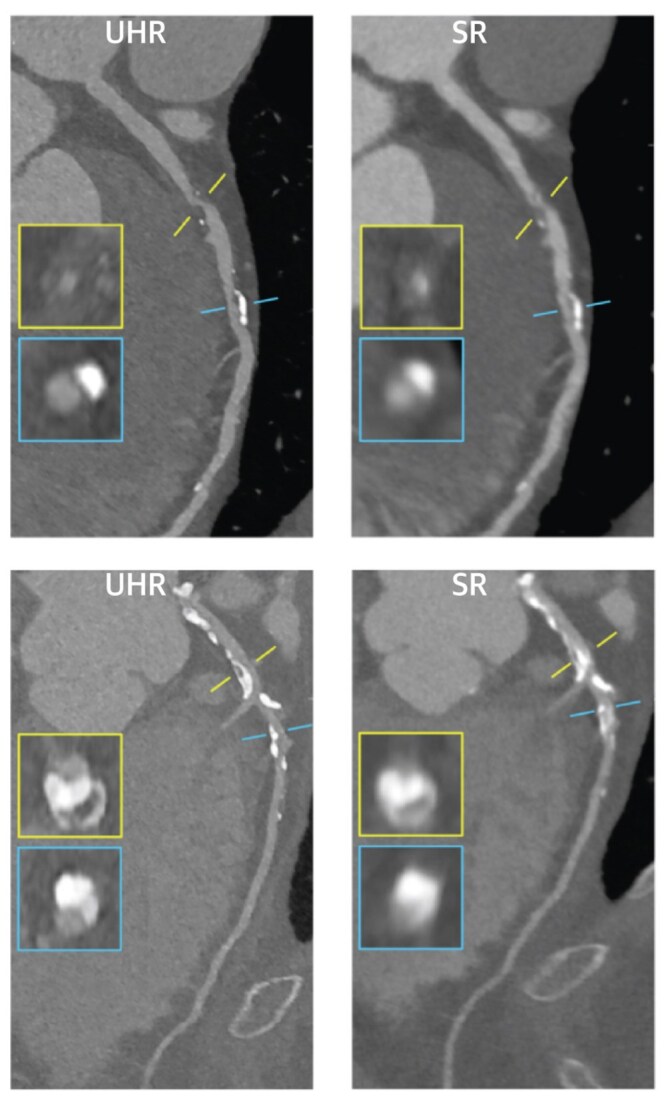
The left anterior descending artery in two patients (upper and lower) demonstrating differences in image readability between ultra-high resolution vs standard resolution photon counting detector CT scans. Ultra-high resolution (UHR); standard resolution (SR). Adapted and reproduced from Kotronias et al.^13^ under a creative commons license.
